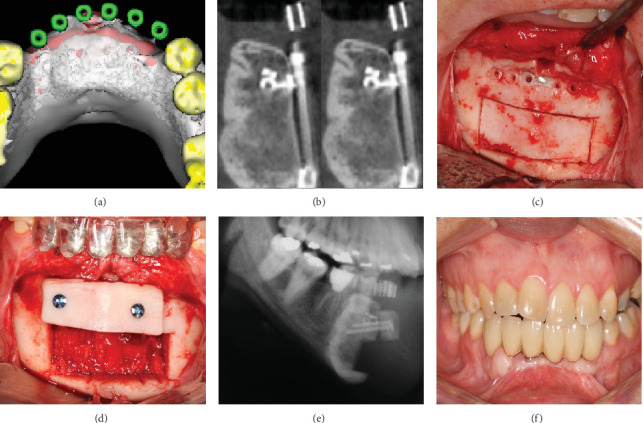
(a) 3D reconstruction planning before implant placement. (b) Autogenous bone graft harvested from the mandibular anterior arch after distractor removal. (c) Bone graft placed near the crestal area for better posterior implant positioning. (d) CBCT image demonstrating lingual bone growth. (e) Cephalometric X-ray immediately after bone grafting. (f) Clinical image of the crowns after the 15-year follow-up.
